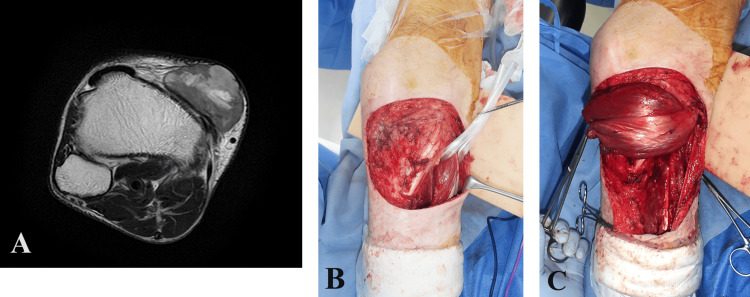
(A) Magnetic resonance imaging revealed a mass located in the proximal anterior compartment of the leg. (B) Following tumor excision, a portion of the tibia and tendon were exposed. (C) The medial gastrocnemius muscle pedicled flap covered the bone and tendon.
